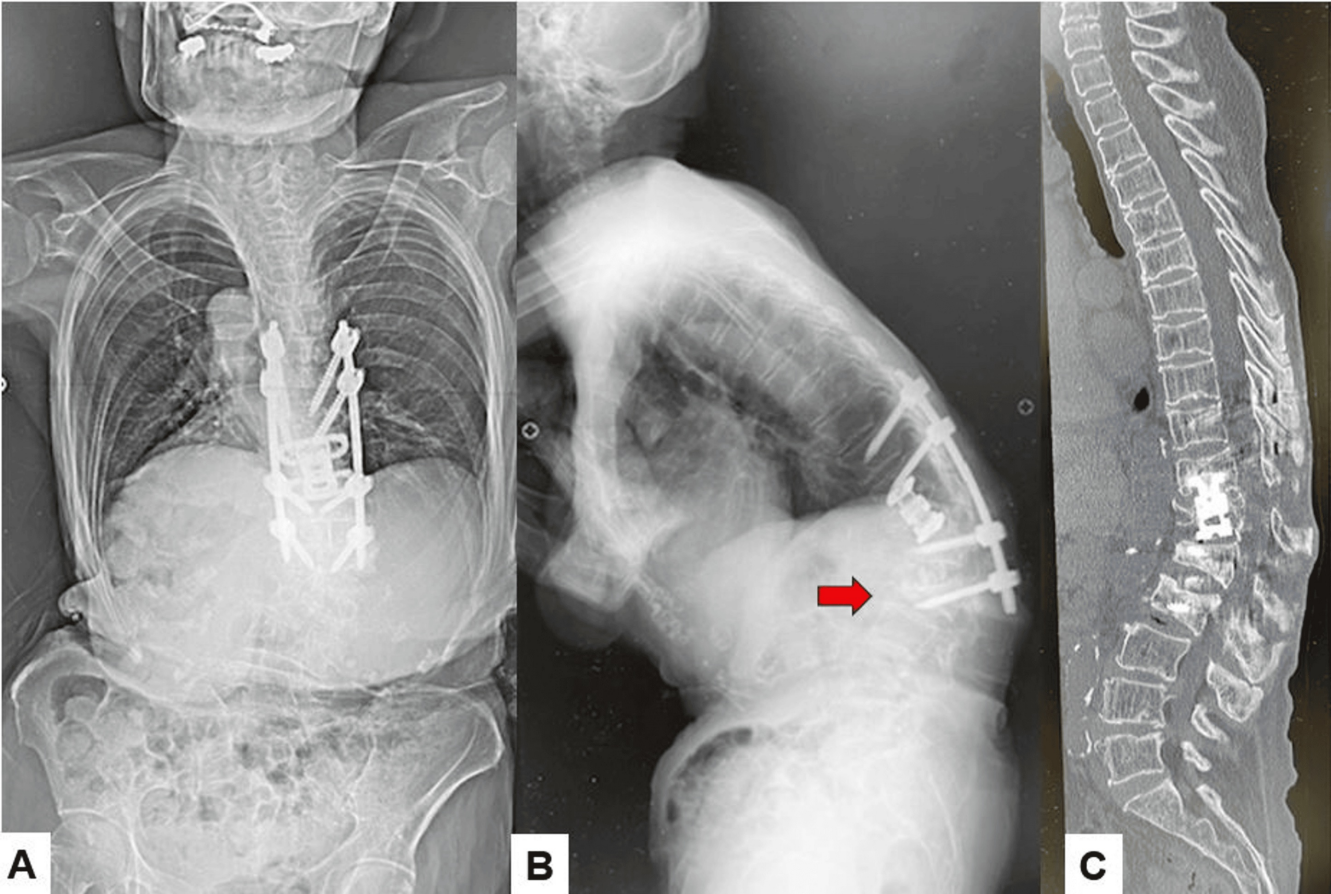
Follow-up images A: Posteroanterior whole spine radiogram, B: Lateral whole spine radiogram, and C: Mid-sagittal reconstruction CT. The red arrow indicates DJK due to a vertebral fracture. DJK: Distal junctional kyphosis
